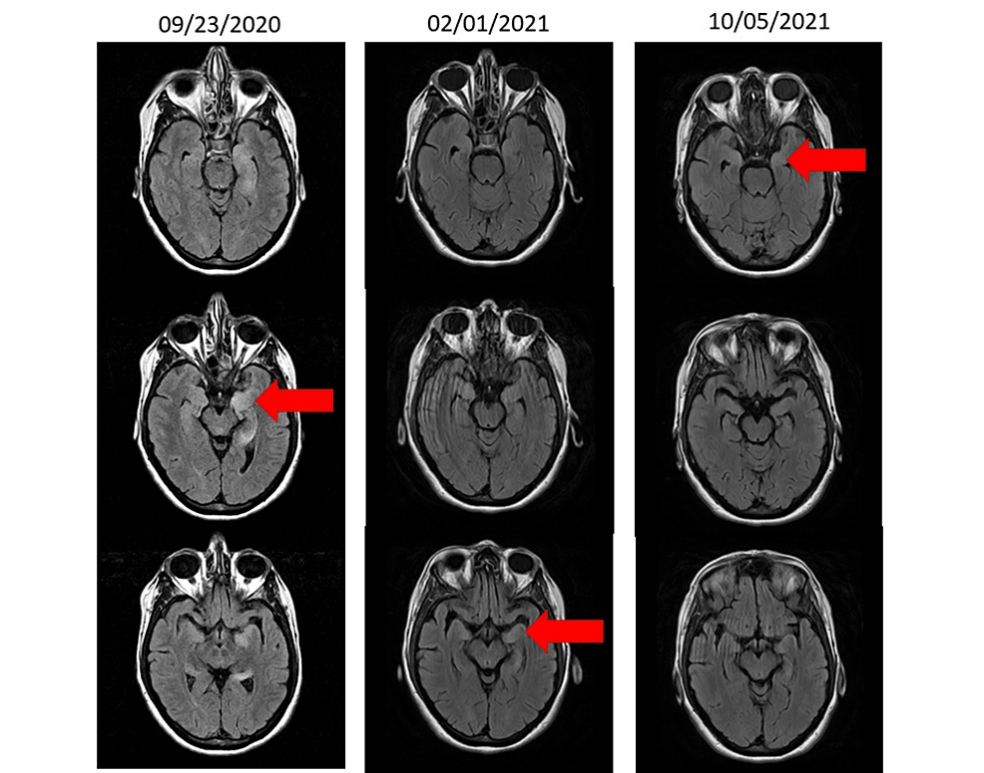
Serial brain magnetic resonance imaging (MRI) T2/FLAIR. 09/23/2020 shows hyperintensity in bilateral mesial temporal lobes, greater on the left side involving the hippocampus and fornix. 02/01/2021 shows subtle FLAIR hyperintensity in medial temporal lobes is mildly reduced compared with prior November imaging. 10/05/2021 shows mild residual T2 signal abnormality amygdala and hippocampi consistent with gliosis related to prior encephalitis. FLAIR, fluid-attenuated inversion recovery
